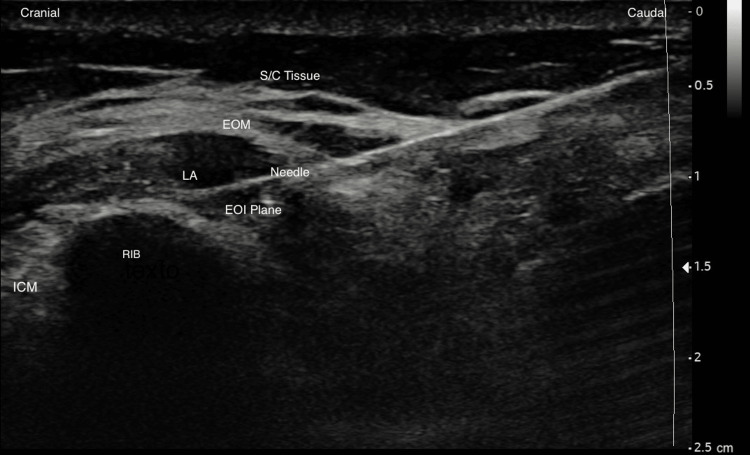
Ultrasound image of the external oblique intercostal plane block ICM: intercostal muscle; EOI: external oblique intercostal; EOM: external oblique muscle; S/C: subcutaneous; LA: local anaesthetic.
